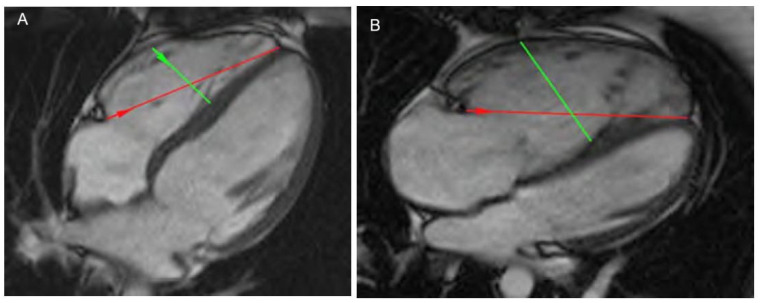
(A) Computed tomography scan of normal heart. (B) Computed tomography scan in porto-pulmonary hypertension showing cardiomegaly with right heart enlargement. Green arrows: Mid-Cavity diameter; Red arrows: Longitudinal diameter.
